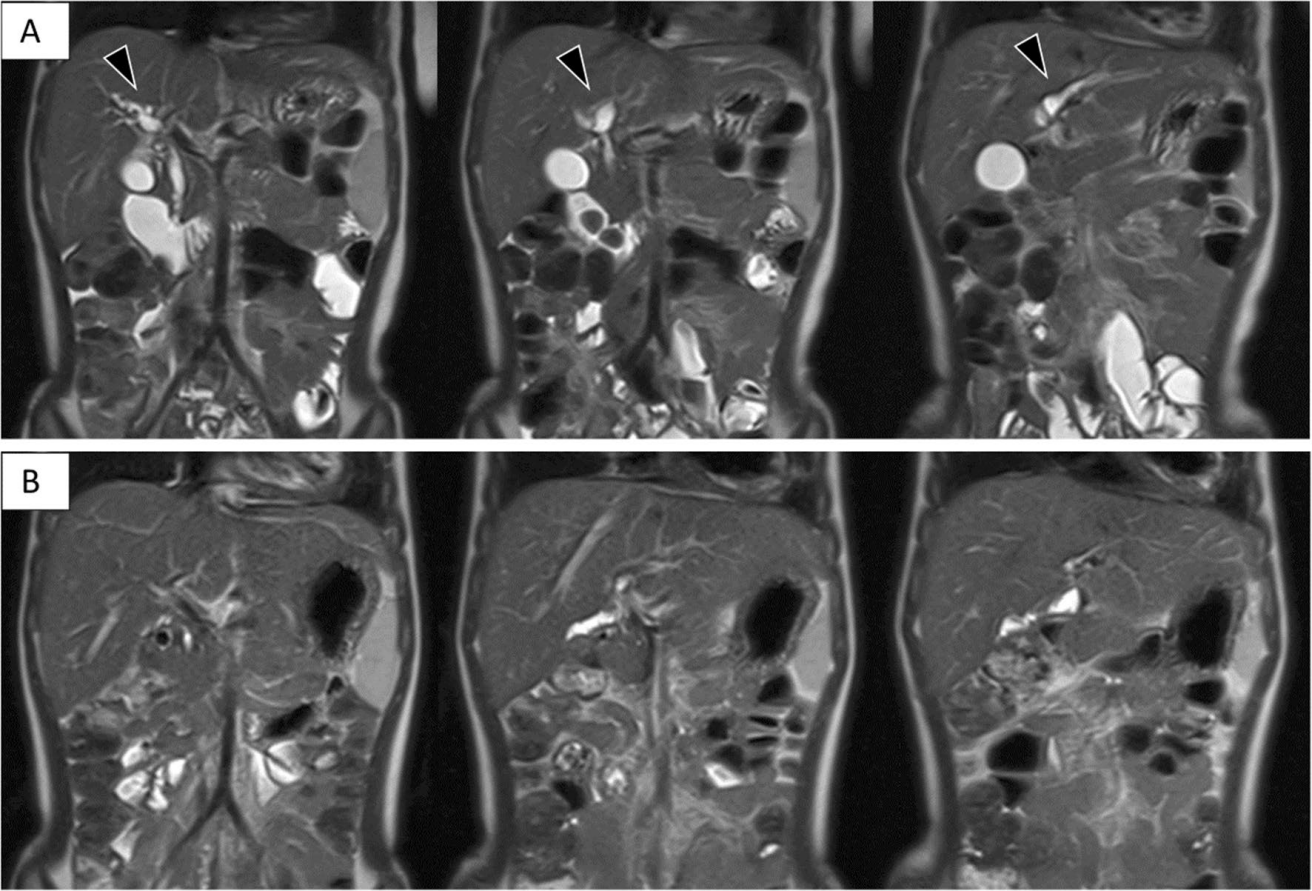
T2-weighted magnetic resonance imaging before and after choledochojejunostomy. A Intrahepatic bile duct dilatation before surgery (arrowhead). B Resolution of intrahepatic bile duct dilatation after surgery
